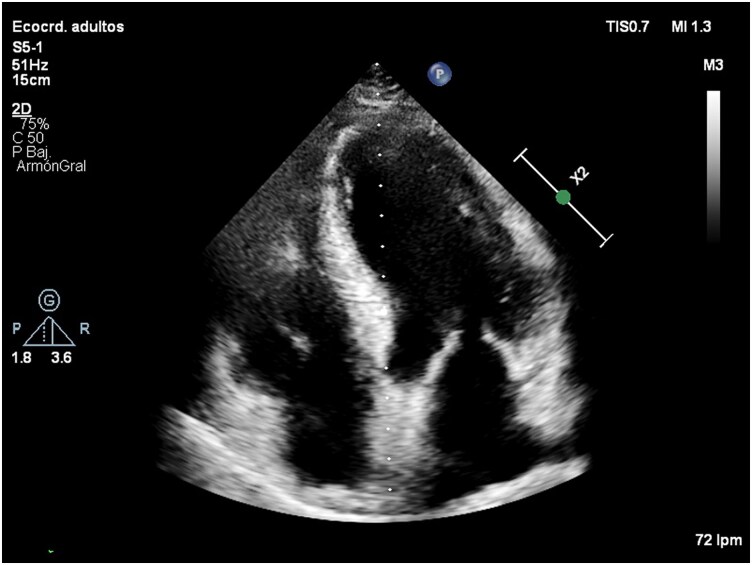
Follow-up transthoracic echocardiogram after surgical repair. Four-chamber apical view. Improved global systolic function with LVEF of 58%.
